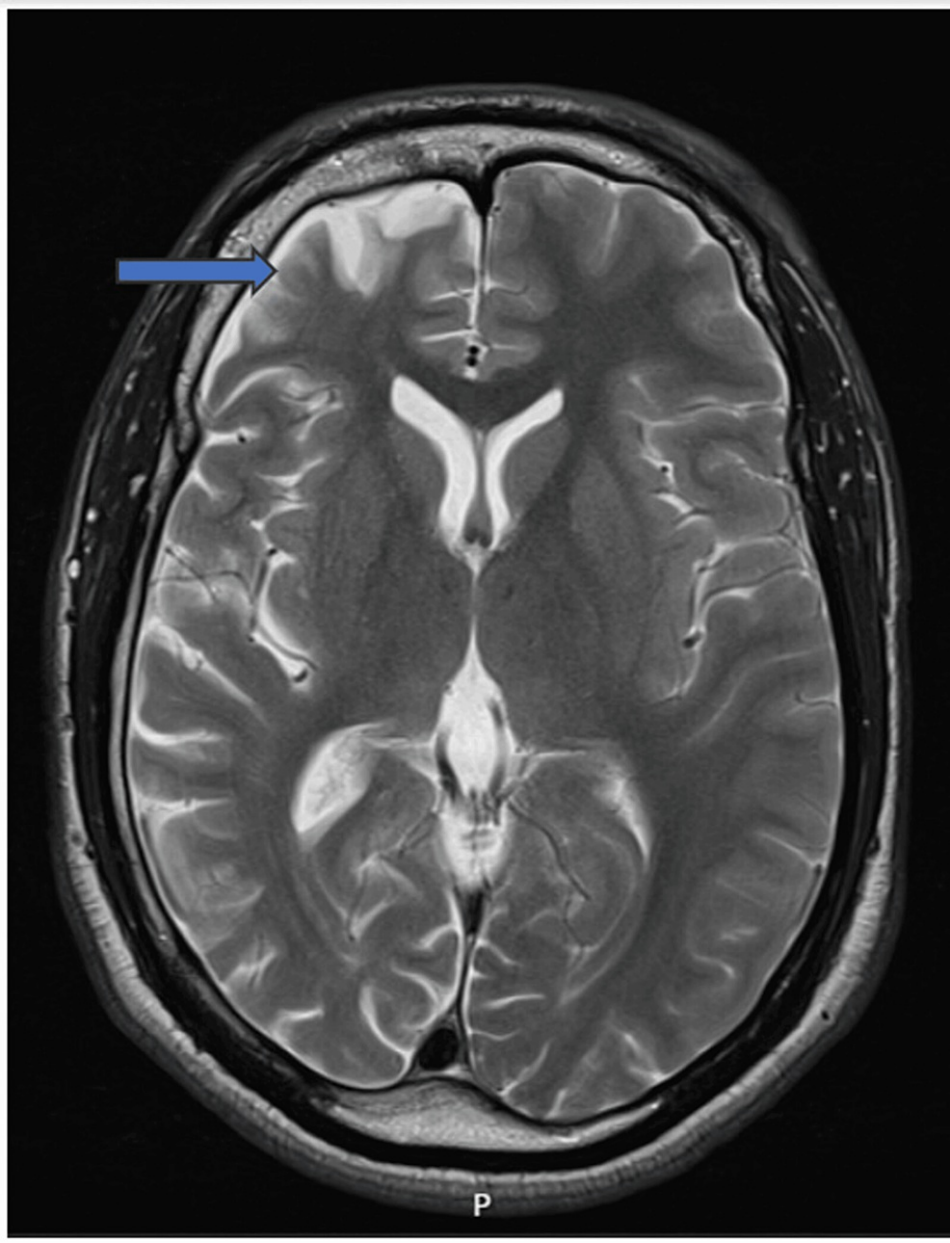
MRI of the head done at age 13 revealing a suggestion of some volume loss in the right frontal lobe with thin gyri and prominent wide sulci and possible gliosis.
